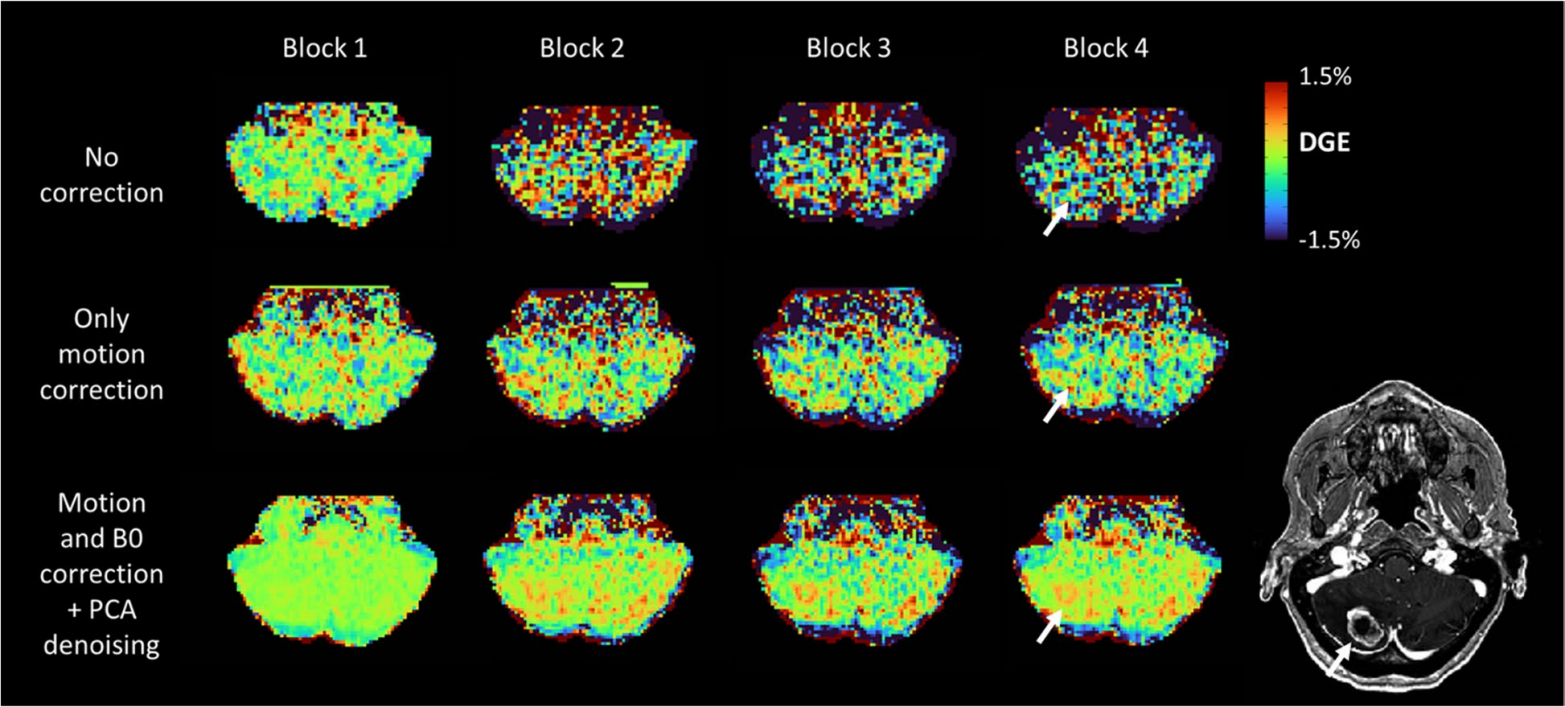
Example of DGE maps (2 ppm) for block 1 through 4 for patient 2, indicating the overall improvement of DGE map quality from no correction (top row), only motion correction (middle row), and with including *B*_0_ correction and principal component analysis denoising (bottom row). Axial slice through the cerebellum, containing enhancing tumor, as depicted in the axial view of the postcontrast T1-weighted image (bottom right). *DGE* Dynamic glucose-enhanced
